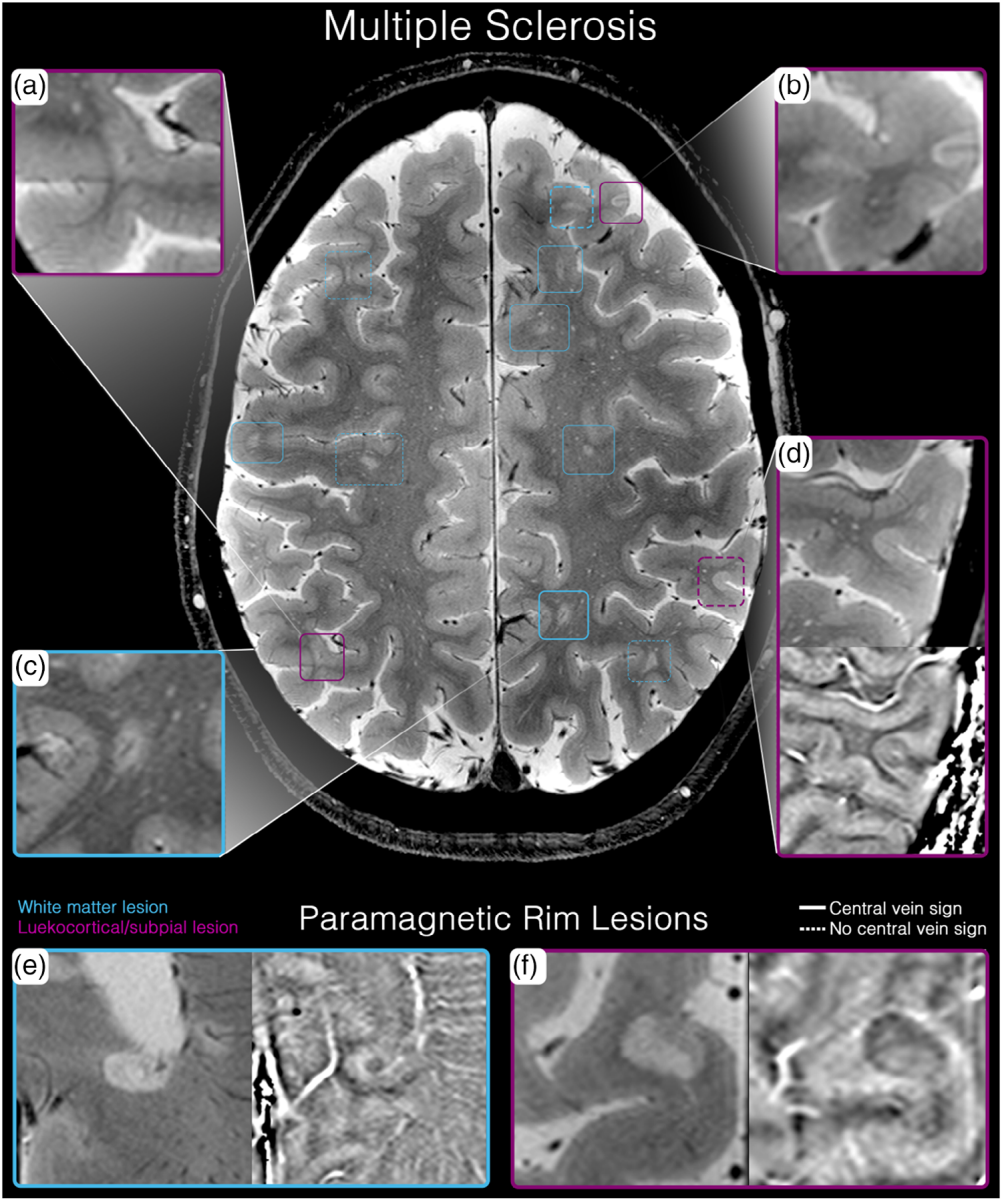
Multiple sclerosis white matter and gray matter lesions using clinical 7 T magnetic resonance imaging (MRI) (axial 2D T2*‐weighted gradient‐recalled echo with a resolution of 0.3 × 0.3 × 0.9 mm^3^: (a) leukocortical (Type I) with a central vein sign; (b) subpial (Type IV) cortical lesion with full cortical coverage and a central vein sign. (c) white matter lesion with a central vein sign; (d) subpial (Type IV) cortical lesion with full cortical coverage, a central vein sign, and a paramagnetic signature; (e) periventricular white matter lesion with a paramagnetic rim and central vein sign; (f) leukocortical (Type I) lesion with a paramagnetic signature.
